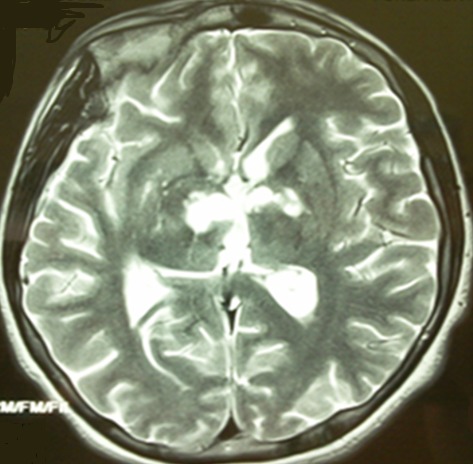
Cerebral MRI axial T2 weighted image: abnormal signal in bilateral anterior thalami and right capsular genu
